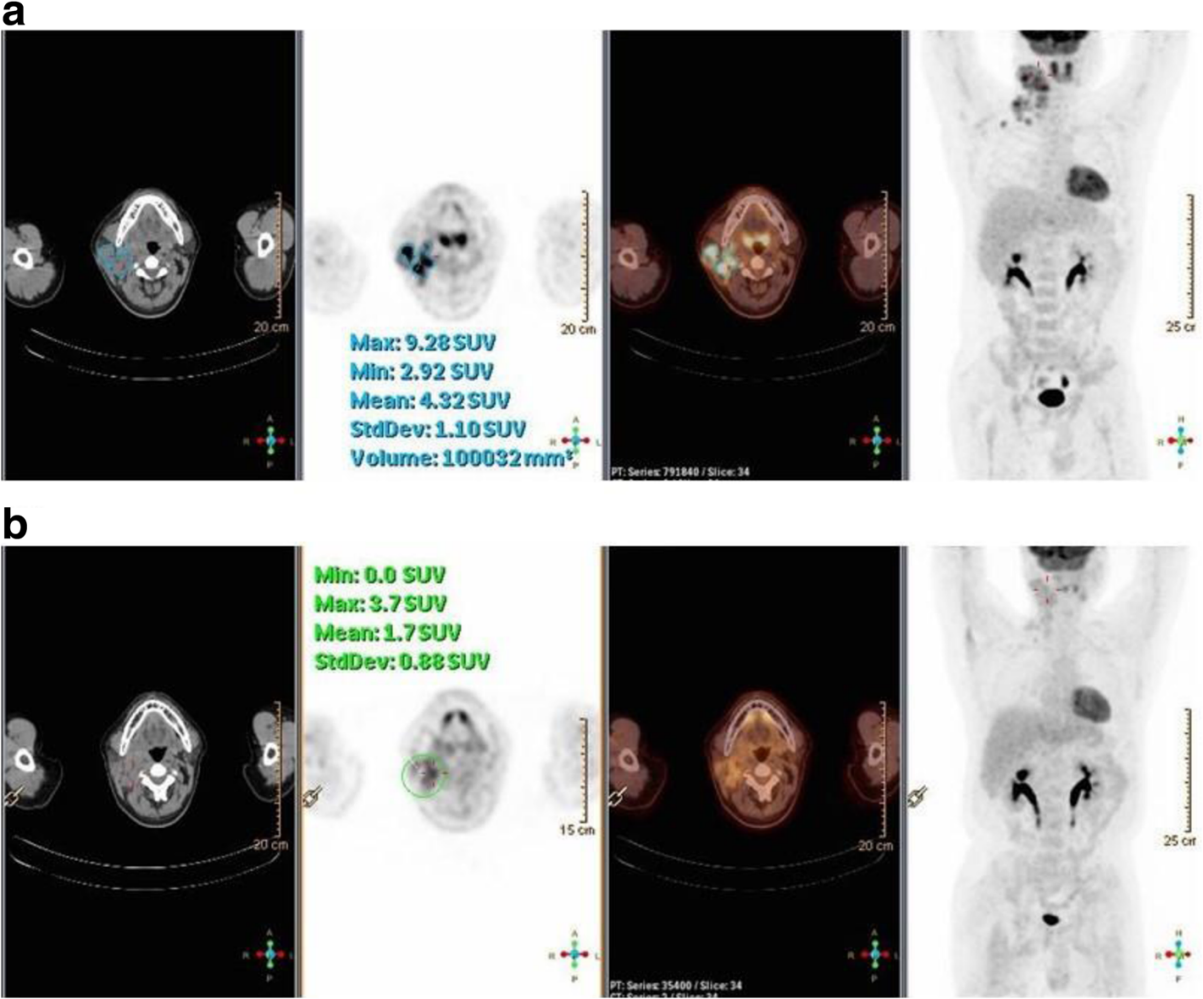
Results of PET/CT scan pre and post anti-PD1 treatment. a The images showed hypermetabolic lesions in right cervical, supraclavicular, axillary and interpectoral lymph nodes before anti-PD1 treatment (baseline scan). b The images showed the lesions were metabolically less active (score 3 on 5-PS) after 3 cycles of anti-PD1 treatment, which indicated that the patient acquired a complete metabolic response
